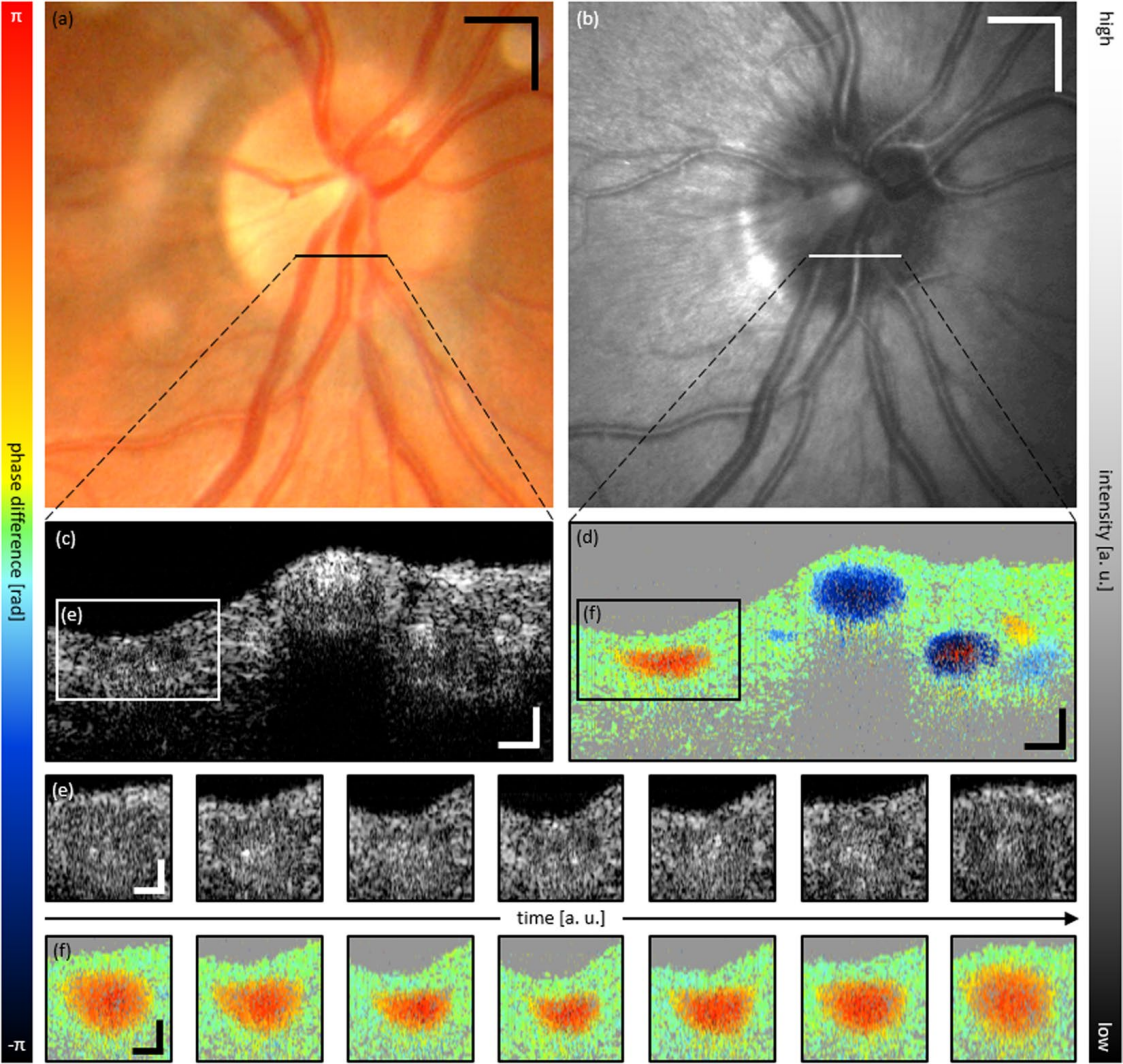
Representative multi-modal image data of SRVP of subject 2. (a) Fundus photography and (b) SLO image with indicated region of collapse. Cross-sectional OCT (c) intensity and (d) Doppler tomogram at indicated region of collapse. (e,f) respective time sequence over full cardiac cycle of ROI indicated in (c,d). Supplementary Video S4 provides a movie-file of the snapshot images of (c,d). Scale bars: (a,b): 0.5 mm; (c–f): 0.1 mm.
